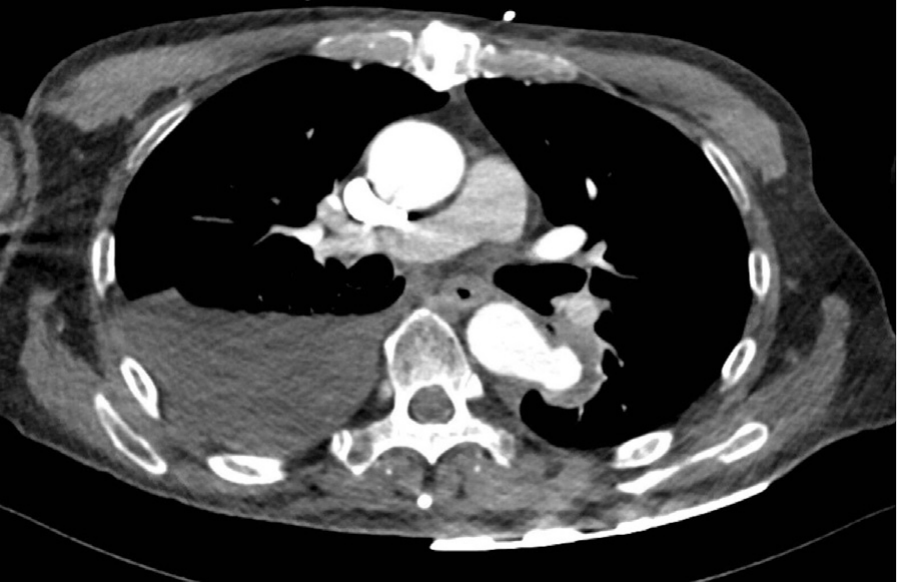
Computed tomography angiography (CTA) displaying axial view of a saccular, 2.4 × 2.9 × 2.5 cm, mycotic aneurysm of the descending thoracic aorta. The aneurysm neck was ∼1.4 cm, and an 8-mm outer portion was thrombosed.
